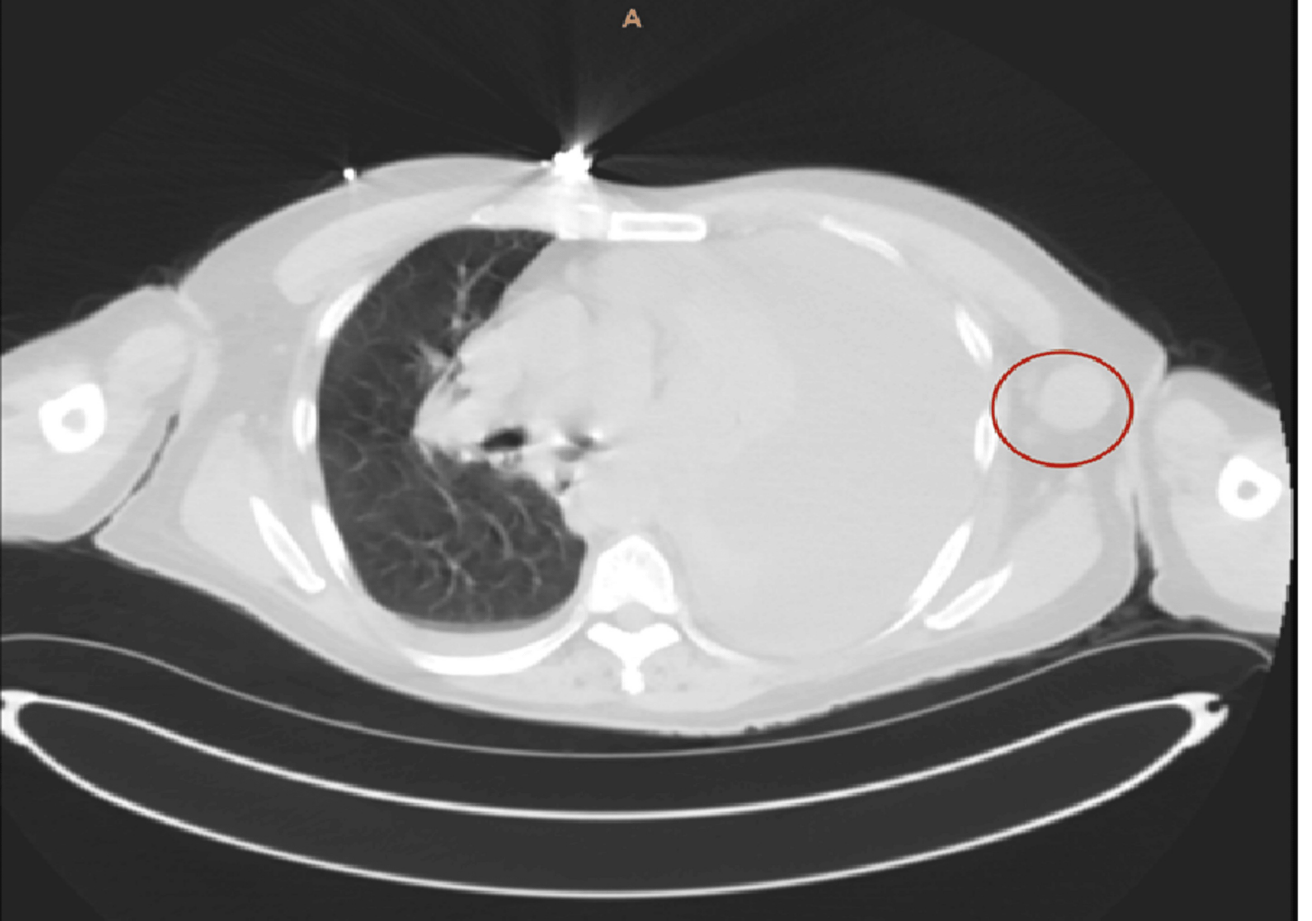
CT chest without contrast Prominent bilateral axillary lymph nodes, more pronounced on the left. A representative left axillary lymph node measures 2.3 cm in diameter (red circle). A left pleural effusion is also noted. CT: computed tomography
